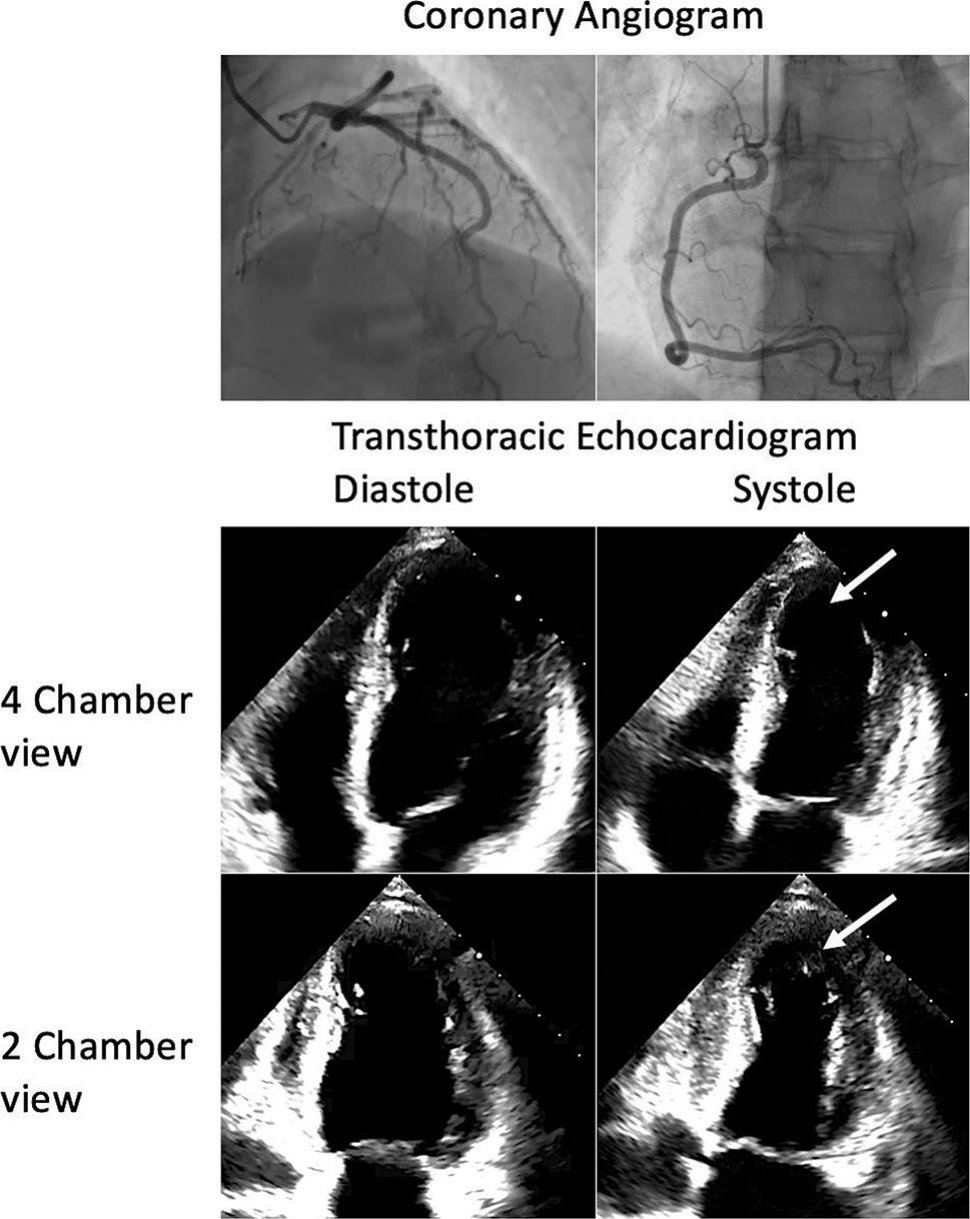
Unobstructed coronary arteries seen on a coronary angiogram (top panel) and a transthoracic echocardiogram demonstrating apical akinesis (white arrows). These findings confirm a diagnosis of conventional apical Takotsubo cardiomyopathy
